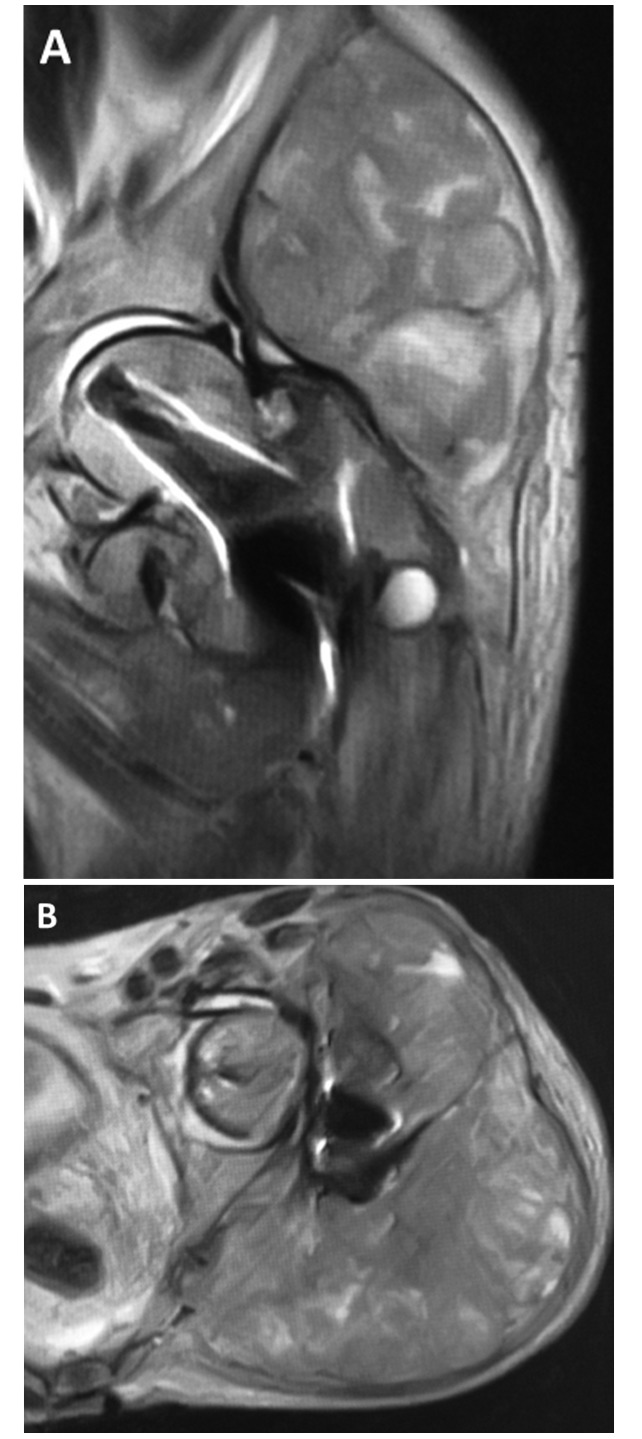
Case one: Magnetic resonance imaging of the left hip. (A) Coronal and (B) axial T2-weighted imaging of the left hip revealing a large mass extending from the femoral neck to the buttock, demonstrating heterogeneous signal hypo- and hyperintensity.
